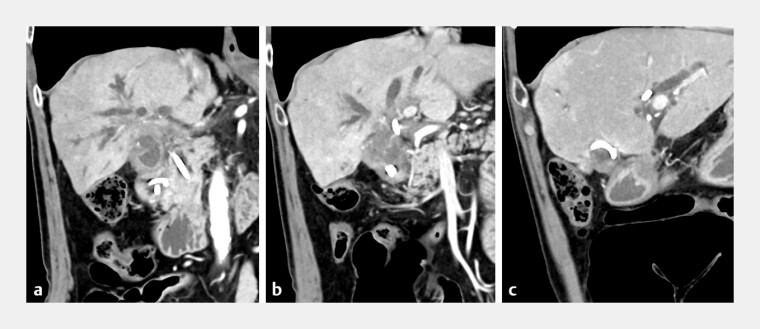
Computed tomography images. A 72-year-old man with malignant hilar obstruction due to gallbladder cancer was admitted to our institution for acute cholangitis associated with recurrent biliary obstruction.
